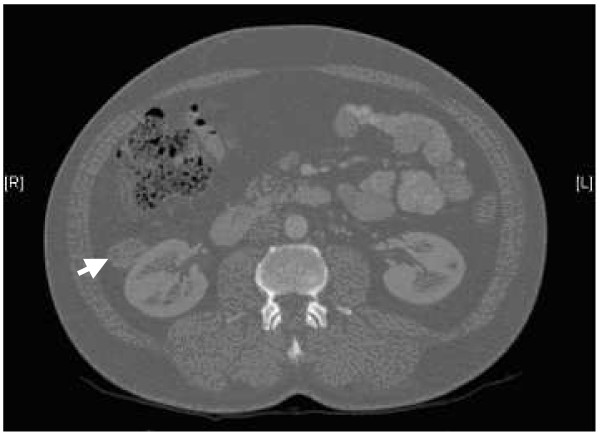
A computed tomography scan showing a right renal mass present on the ventral aspect of the kidney away from the hilar region (arrow).
